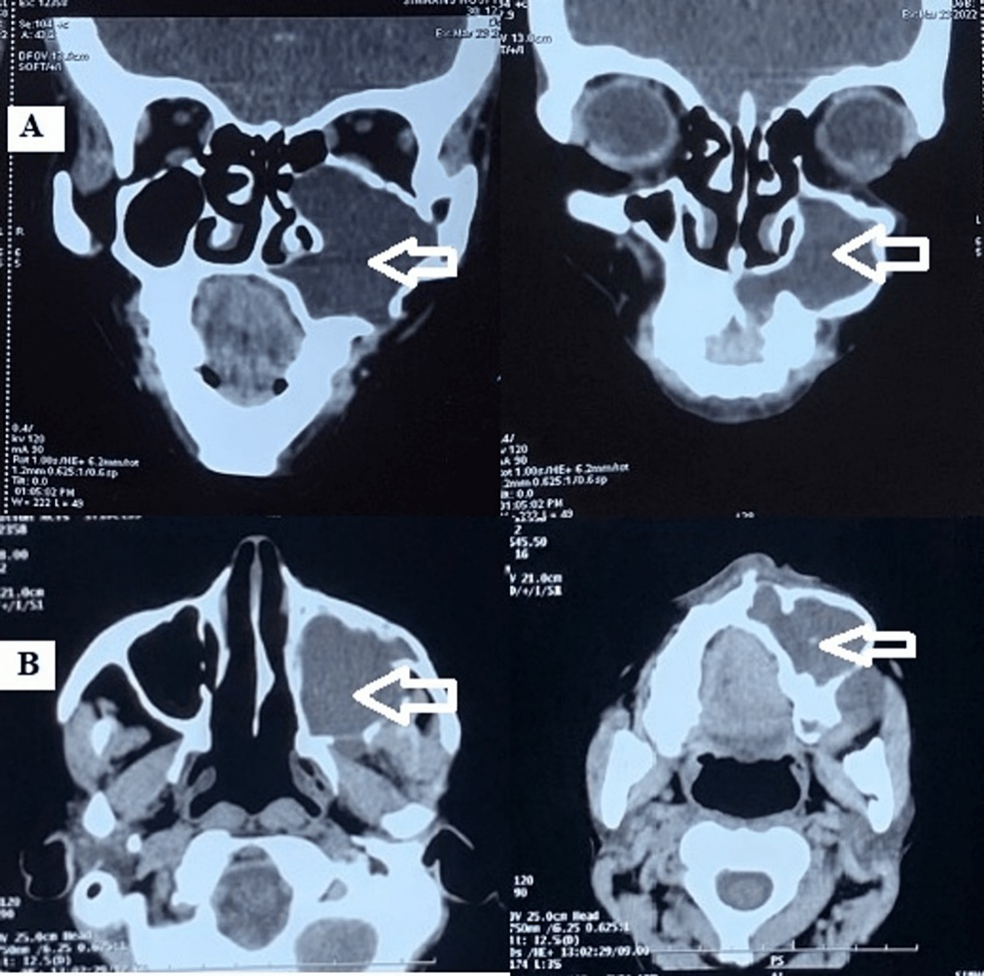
(A) Coronal and (B) axial CT: the expansile lytic lesion in the left alveolus with hypodense non-enhancing soft tissue component
